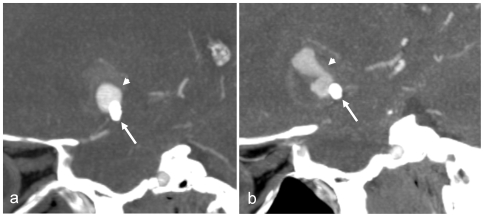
Partially thrombosed aneurysm after treatment with the flow diverter. CT angiograms showing a residual lumen within a large partially thrombosed fusiform aneurysm of the middle cerebral artery. Follow-up CTA (a) was performed 4 months and (b) 6 months after SFD (arrows) placement. The second follow-up study shows enlargement of the residual aneurysm lumen (arrow heads) and was performed after a new haemorrhage (not shown).
